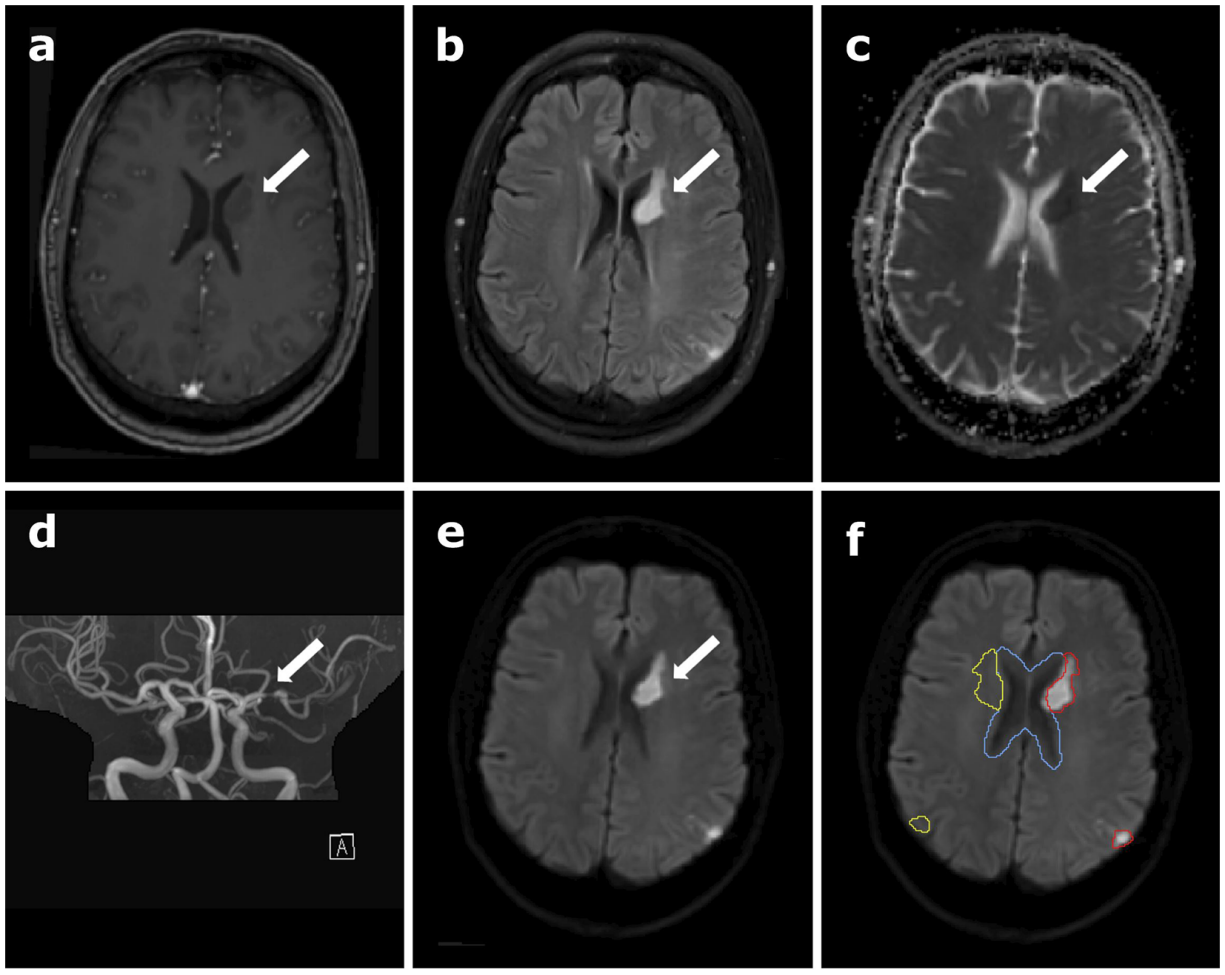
Clinical data of the patient depicting the stroke region, (A) contrast-enhanced T1-weighted magnetization prepared rapid gradient echo (MPRAGE) data, (B) T2-weighted fluid-attenuated inversion recovery (T2 FLAIR), (C) apparent diffusion coefficient (ADC) map calculated from diffusion-weighted imaging (DWI), (D) time-of-flight angiography (TOF), (E) B1000 DWI, and (F) B1000 DWI overlaid with the different regions of interest (ROI) used for data analysis in oxygen-17 (^17^O) MR data: the red contour outlines the stroke region, and cerebral spinal fluid (CSF) is delineated in blue. The yellow contour shows the mirrored stroke ROI, comprising healthy brain tissue. The white arrows indicate the location of the stroke.
